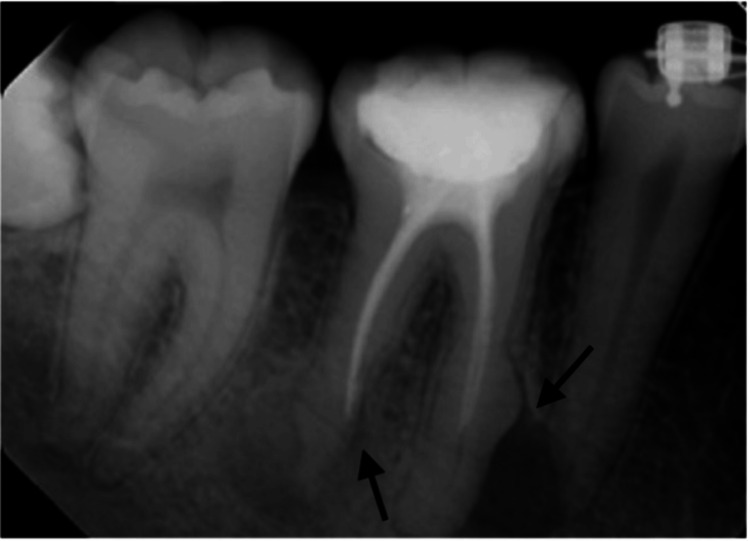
Preoperative periapical radiograph showing periapical lesions with mesial hypercementosis and external root resorption of the distal root.
